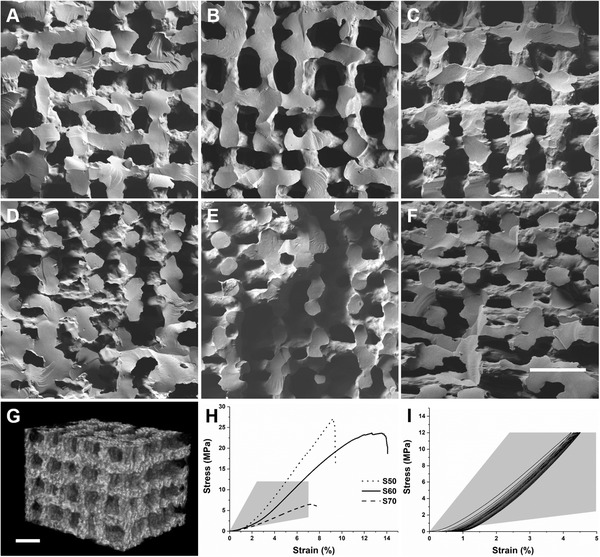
SEM images of horizontal cross‐sections of 3D printed hybrid scaffolds: A) S50, B) S60, and C) S70 (the numbers in S50, S60, and S70 refer to organic wt%). SEM images of vertical cross‐sections of D) S50, E) S60, and F) S70. G) Representative 3D micro‐CT image of the central region of S60 (scale bars: 500 µm). H) Representative uniaxial stress/strain curves of the 3D printed scaffolds. I) Cyclic compressive loading stress/strain curve of S60 (mechanical property region of trabecular bone^[^
